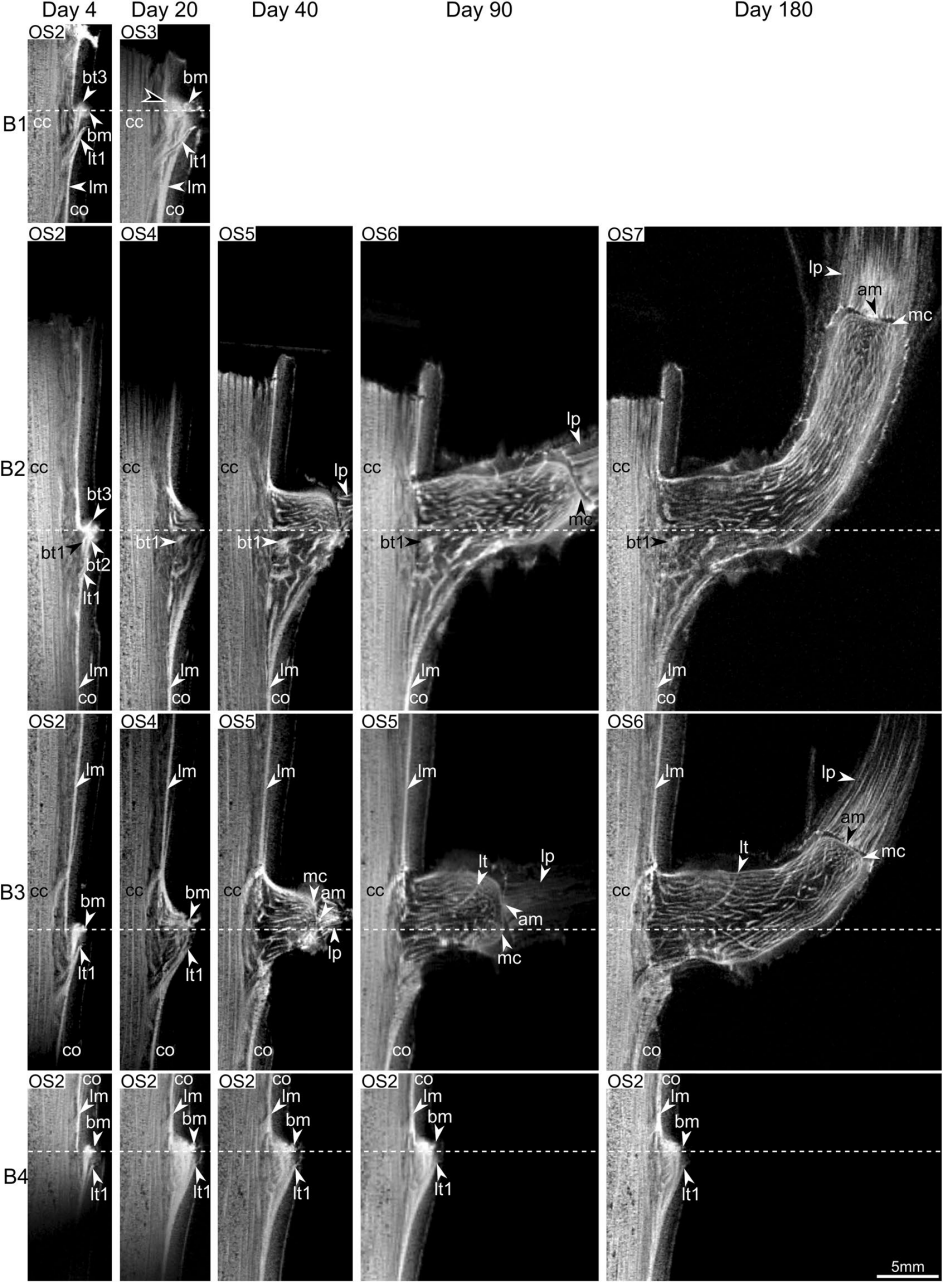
Sagittal MR images of the bud ontogeny in *Dracaena marginata*. Rows: images of buds B1–B4 respectively. Columns: day 4-day 180 of image acquisition after decapitation. Bud B1 died back after 25 days and is therefore missing in the columns day 40-day 180. A reference line is placed into the center of the bud meristem (bm), splitting the vascular bundles of the branch into adaxial bundles which run in the branch perpendicular to the main stem and abaxial bundles describing a curvature arrangement from the main stem and into the branch towards the branch apex. The ontogenetic stage (OS2–OS7) for each individual image is given. The white framed arrow in the image of B1 at day 20 highlights the fusion of bud meristem (bm) and lateral meristem (lm). am: apical meristem; bm: bud meristem; bt1–3: specific bud traces (also see Fig. 3); cc: central cylinder; co: cortex; lm: lateral meristem; lp: leaf primordium; lt: leaf trace; lt1; specific leaf trace; mc: meristematic cap.
